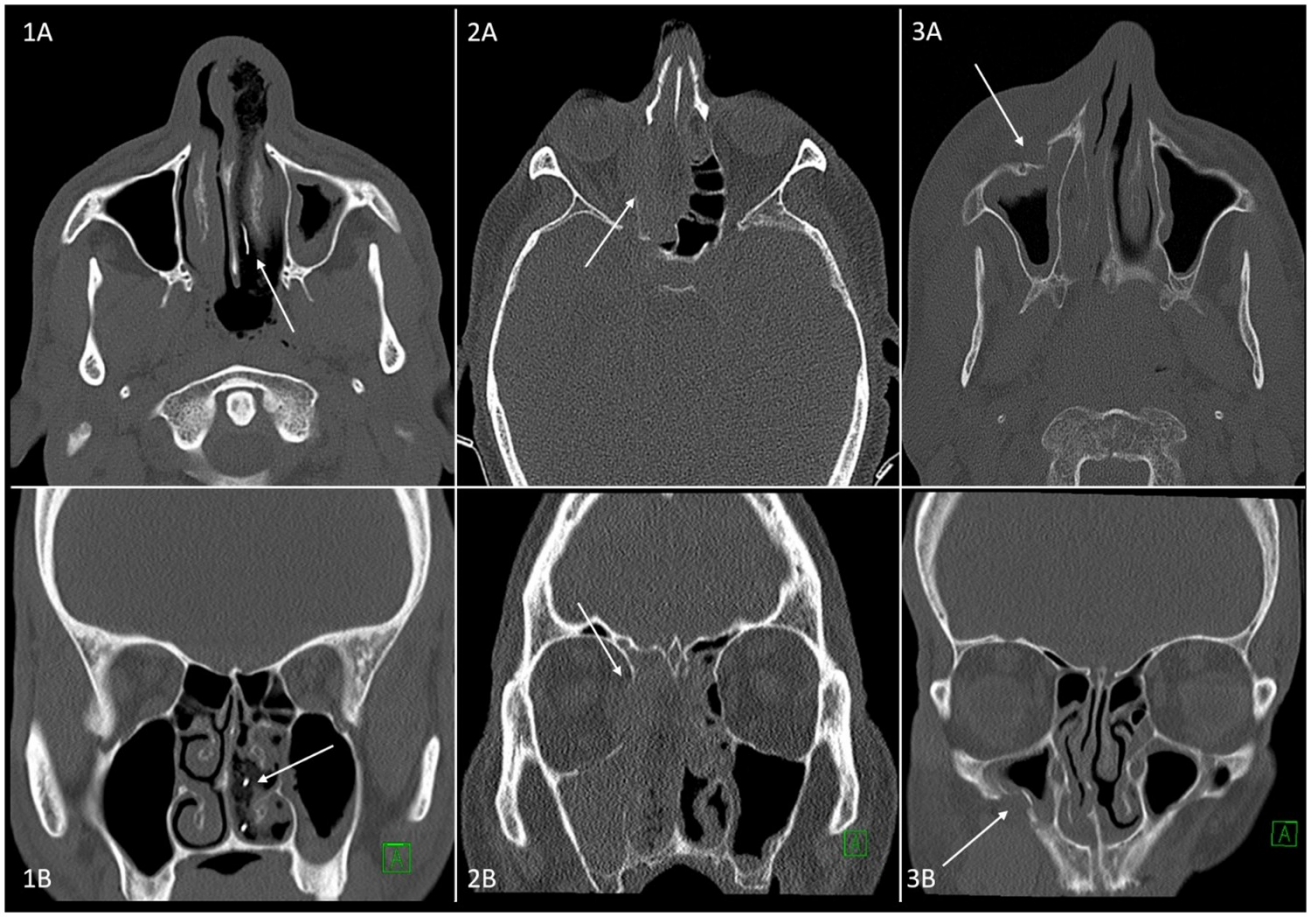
Examples of typical imaging findings in CT. Fig 1 displays examinations of non-contrast computed tomography of paranasal sinuses (CT) of 3 different patients (numbered 1–3), each with axial (upper row) and coronal (lower row) reconstructions. In column 1, white arrows indicate a nasal tamponade in a patient with SE due to bleeding of sphenopalatine artery. In column 2, white arrows indicate a tumorous mass (squamous cell carcinoma) in the nasal cavity, which infiltrates the medial wall of the eye cavity as well as the nasal septum and maxillary sinus. In column 3, arrows indicate a fracture of the anterior wall of the maxillary sinus with consecutive circular swelling of the mucous tissue and epistaxis.
